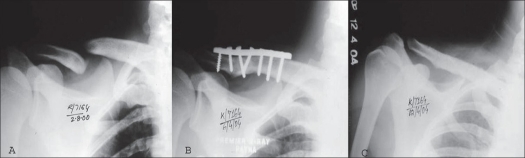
X-ray of shoulder antero- posterior view of a 35 years old male shows, A) 6 months old fracture clavicle. B) Union after DCP and bone grafting. C) After plate removal
